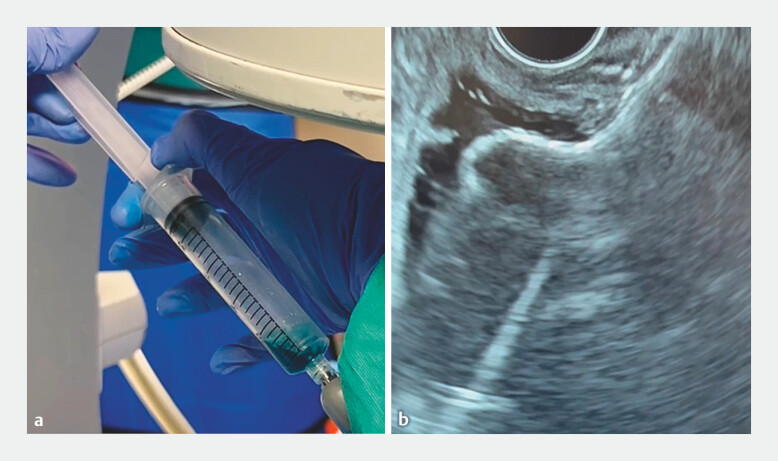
Images during creation of the endoscopic ultrasound-guided entero-enterostomy showing:
